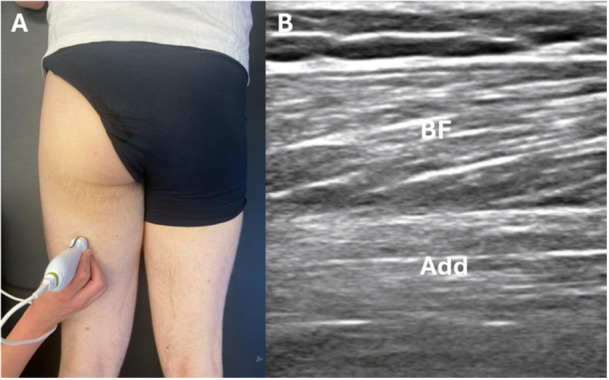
(A) Overview of probe placement, (B) longitudinal ultrasound view of the Biceps femoris (BF: Biceps femoris long head). Add: adductor magnus.
